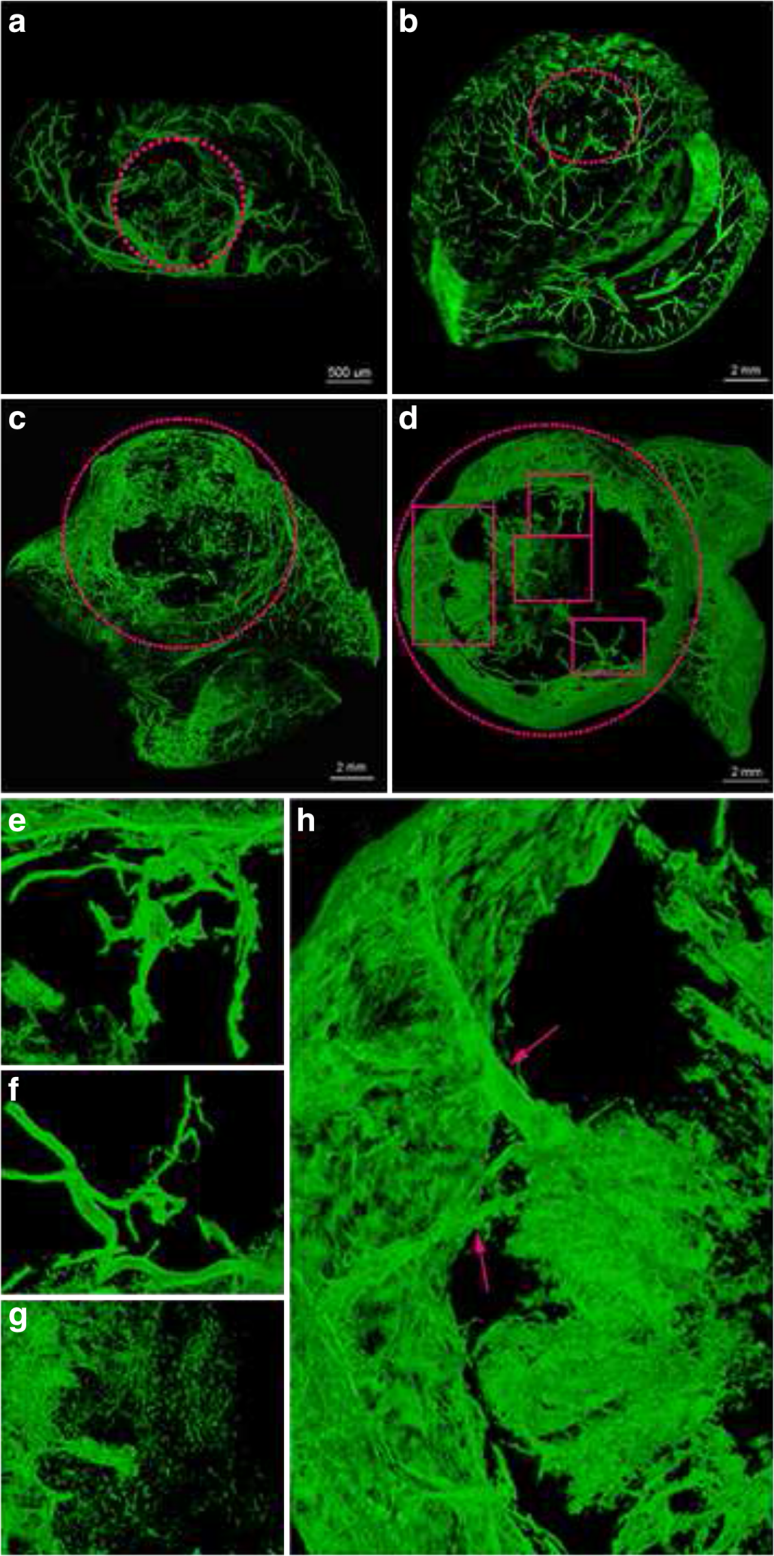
3D reconstruction of contrast CT images for HCC neovascularization at weeks 1 to 4 (a to d, respectively). The selected areas with red dot lines show the tumor regions, (e to f) enlarged image of the rectangle region of d, which clearly shows the disorganized distribution and morphology of tumor neovascularization. A large number of avascular regions were observed in the tumors at weeks 3 and 4 (c, d), as well as irregular vessel shape with dendritic-like branching (e), individual vascular curvature abnormalities (f), blood vessel network cluster structure (g), a large number of tiny and curved vessels derived from a few thick vessels (h, *red arrows*), and compressed tumor edge or peripheral vasculature (c, d)
